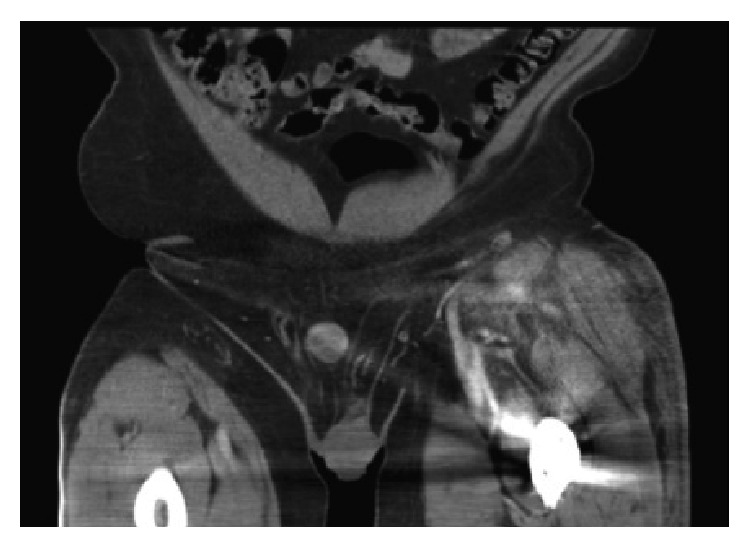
Contrast CT of the left lower extremity showing a suspected pseudoaneurysm of the left profunda femoris artery.
